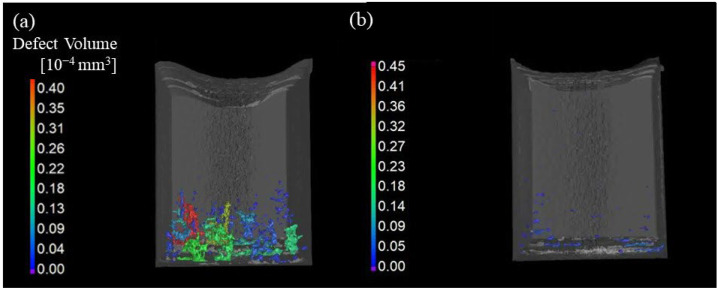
The results of X-ray CT of (a) uninoculated and (b) inoculated SUS316L samples manufactured by the LAMDA 200 machine.
